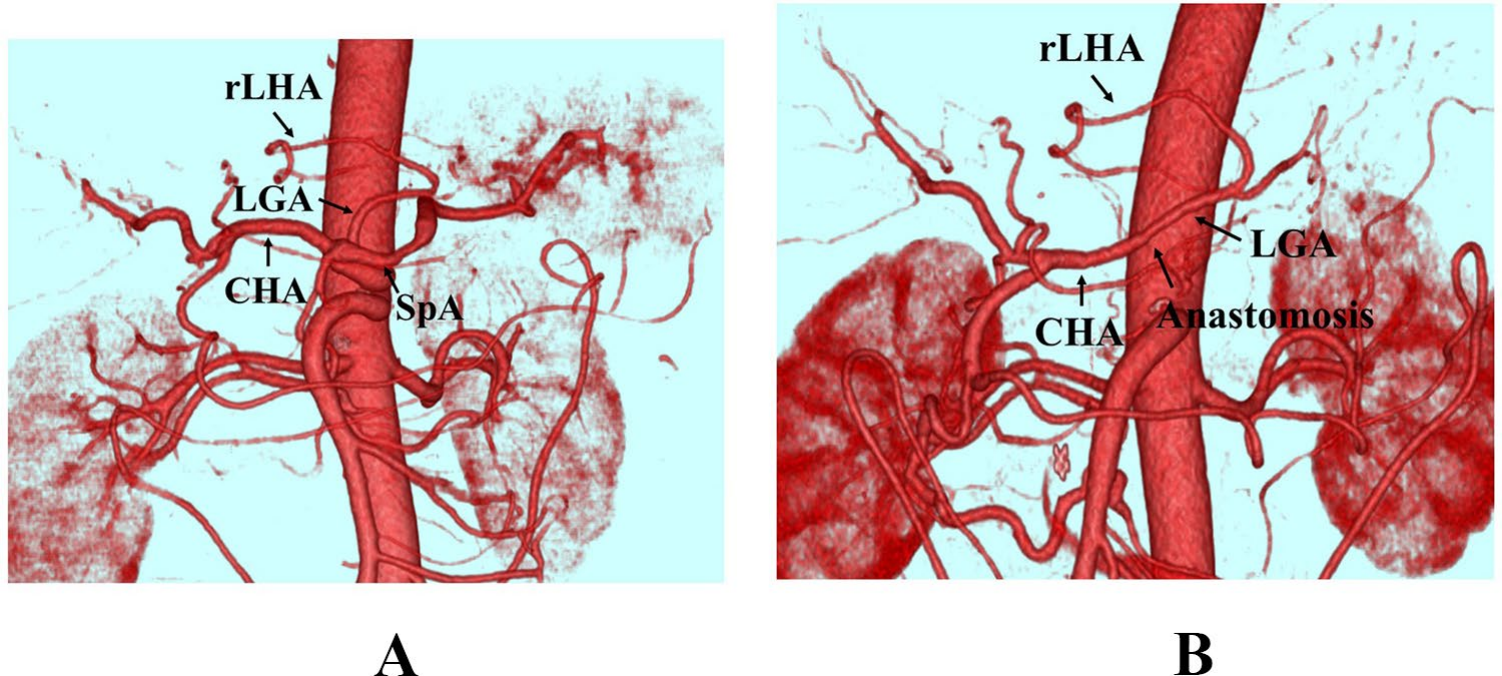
3D computed tomography angiography; A preoperative image showing the replaced left hepatic artery (rLHA) arising the left gastric artery (LGA); B postoperative image showing patent rLHA and LGA from the common hepatic artery; *CHA* common hepatic artery, *LGA* left gastric artery, *rLHA* replaced left hepatic artery, *SpA* splenic artery
